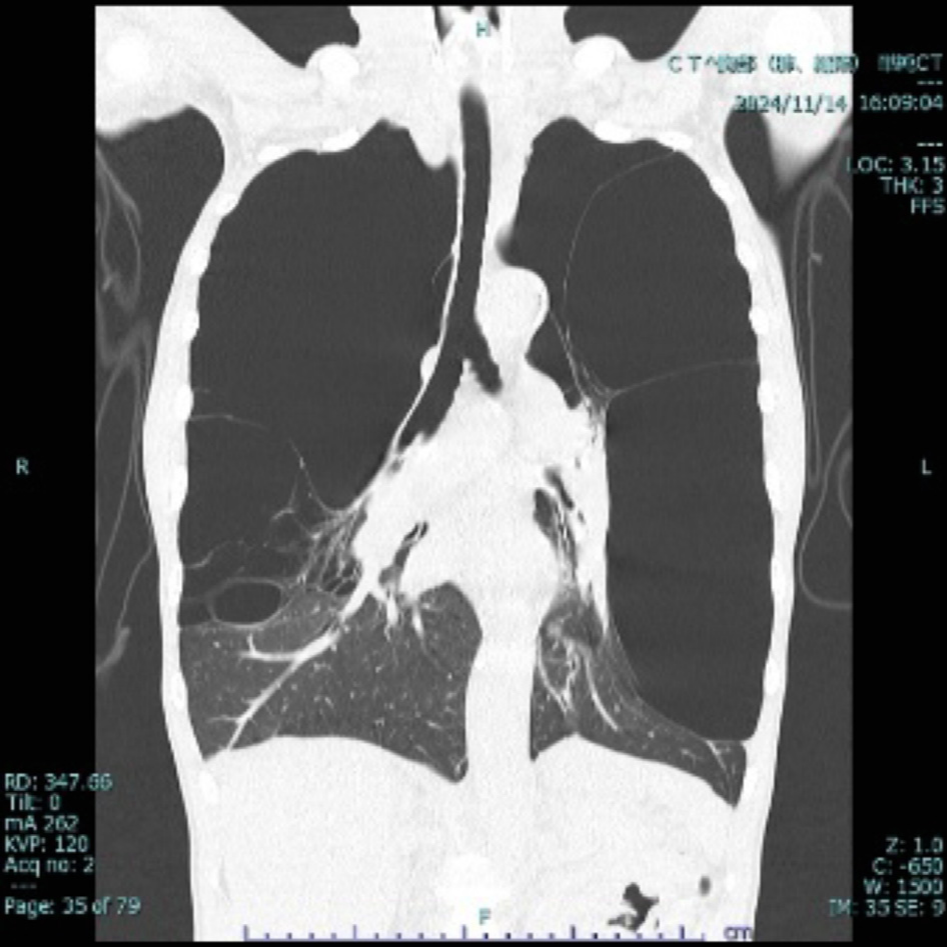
The area of hyperlucency that appeared to be a pneumothorax on chest radiography is found to be a giant bulla on computed tomography.
